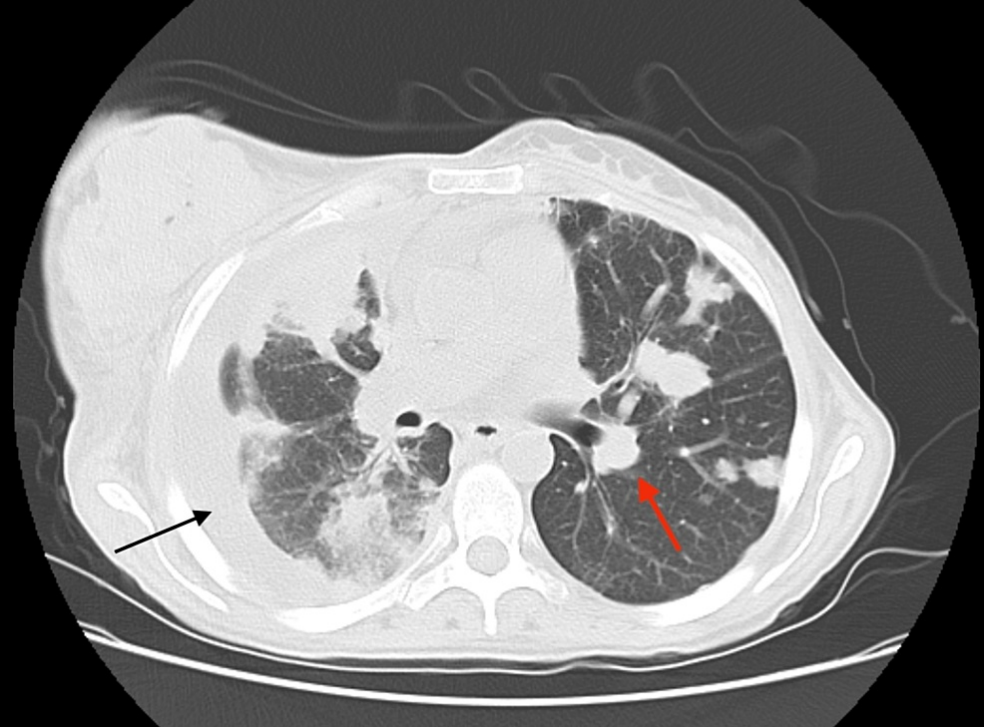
Pleural carcinomatosis and pulmonary metastases Contrast-enhanced thoracic CT showing a loculated right pleural effusion with circumferential irregular pleural thickening (black arrow) and multiple pulmonary nodular lesions with spiculated contours (red arrow).
